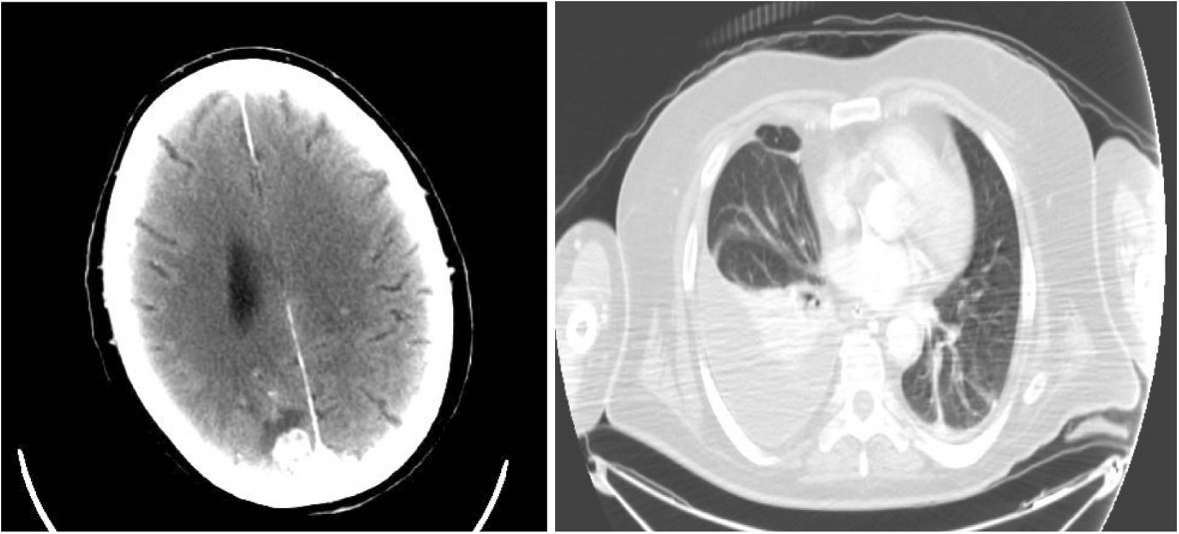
Computed tomography of the brain and thorax.
